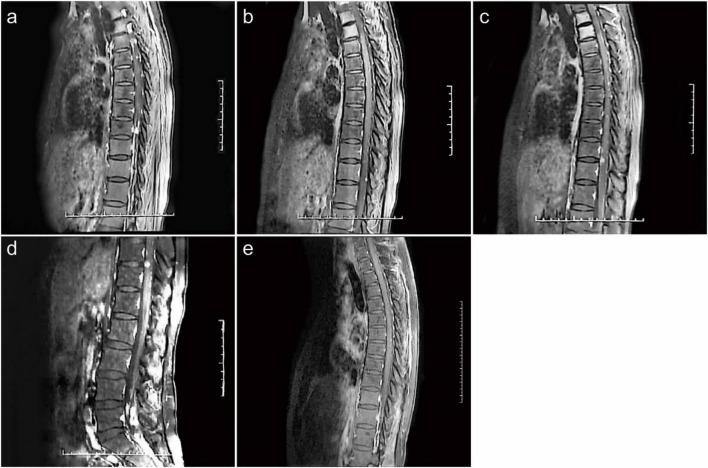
Magnetic resonance imaging (MRI) comparison of thoracic spinal cord metastases before and after treatment. (a–d) Baseline images (obtained from an external hospital in July 2022; photographs of the workstation screen). (a–c) Thoracic spine images showing multiple metastatic lesions. (d) Lumbar spine image, in which a prominent metastatic lesion in the lower thoracic segment is clearly visible. This image is included due to the limited availability of baseline images, to provide a clear baseline reference for comparison. (e) Follow-up image (acquired at our hospital in October 2022), showing marked reduction of the thoracic spinal cord metastases.
